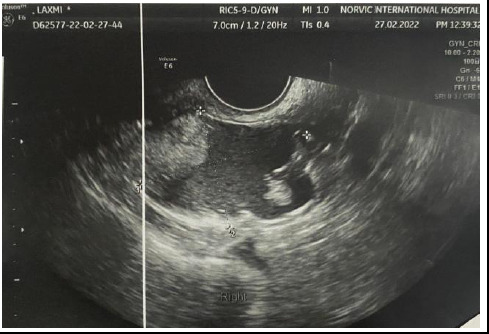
Ultrasonography showing cyst in right ovary measuring 50x35 mm with internal echoes and hyperechoic component, likely a dermoid cyst.
